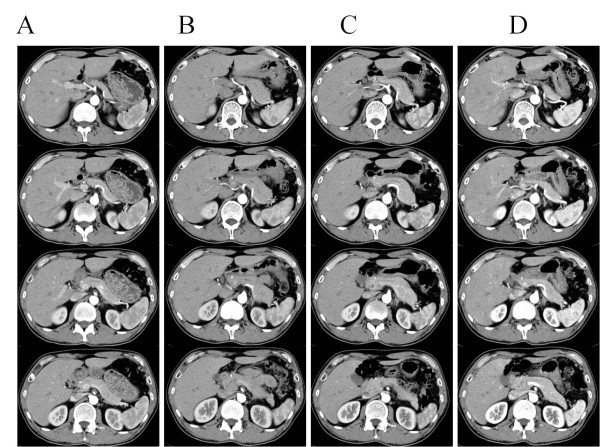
Pancreatic enlargement ((A), first examination; (B-D), two months, three months and one year later, respectively). At two months, computed tomography revealed an enlargement of the pancreatic tail adjacent to the splenic artery. However, this enlargement of the pancreas gradually improved.
